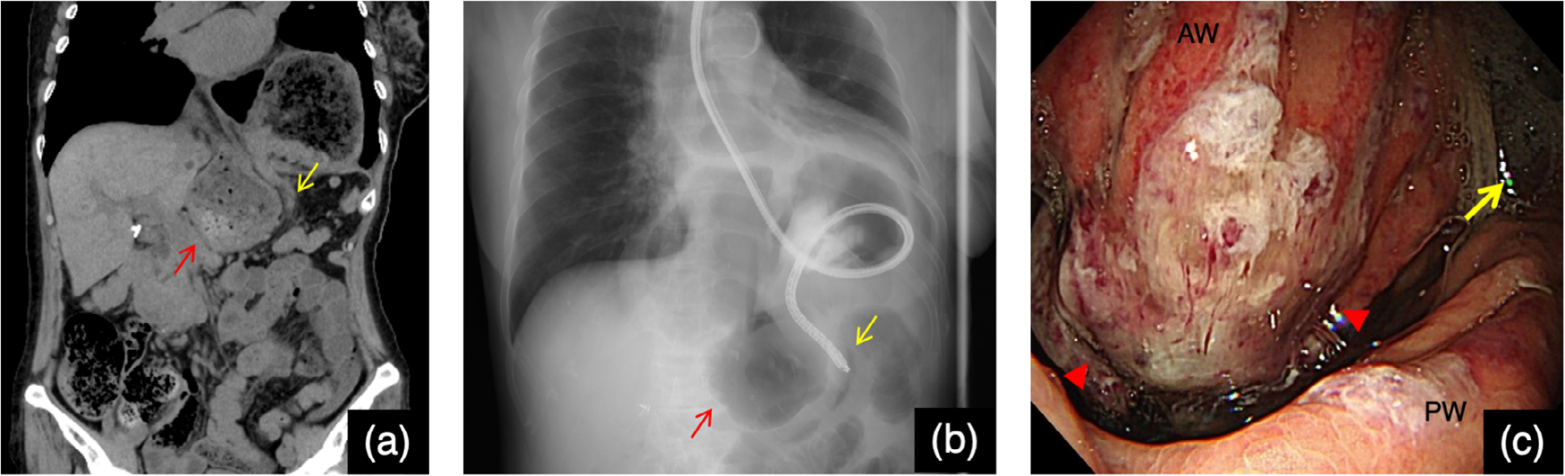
Imaging findings on readmission (a) Computed tomography shows hiatal hernia and incarceration of a part of the gastric body into the abdominal cavity. The red arrow shows the incarceration of a part of the gastric body into the abdominal cavity, and the yellow arrow shows the compressed duodenum. (b) This fluoroscopic image shows the insertion of the esophagogastroduodenoscope into the duodenum to straighten the stomach. The red arrow shows the incarceration of a part of the gastric body into the abdominal cavity, and the yellow arrows show the compressed duodenum. (c) Esophagogastroduodenoscopy detected severe Cameron's ulcer. The red arrowheads show the ulcer. The yellow arrow points in the direction of the gastric antrum. AW: anterior wall, PW: posterior wall.
